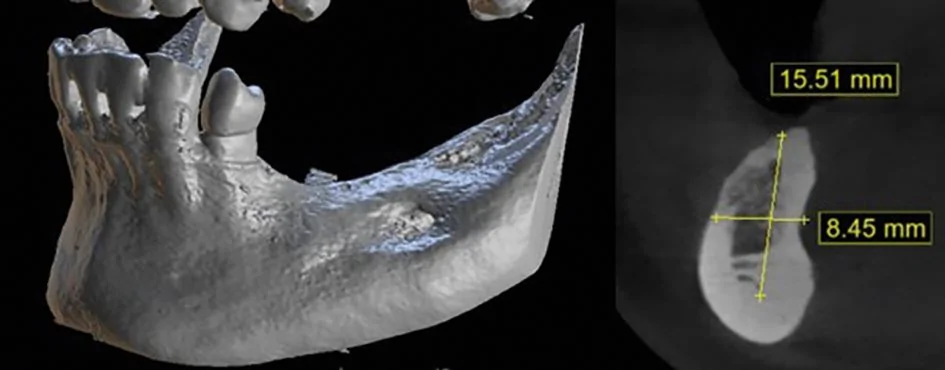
Cone beam computed tomography render of the post-operative situation. The ‘new' mental foramen now posterior from the original mental foramen. The buccal bone has completely integrated and healed
